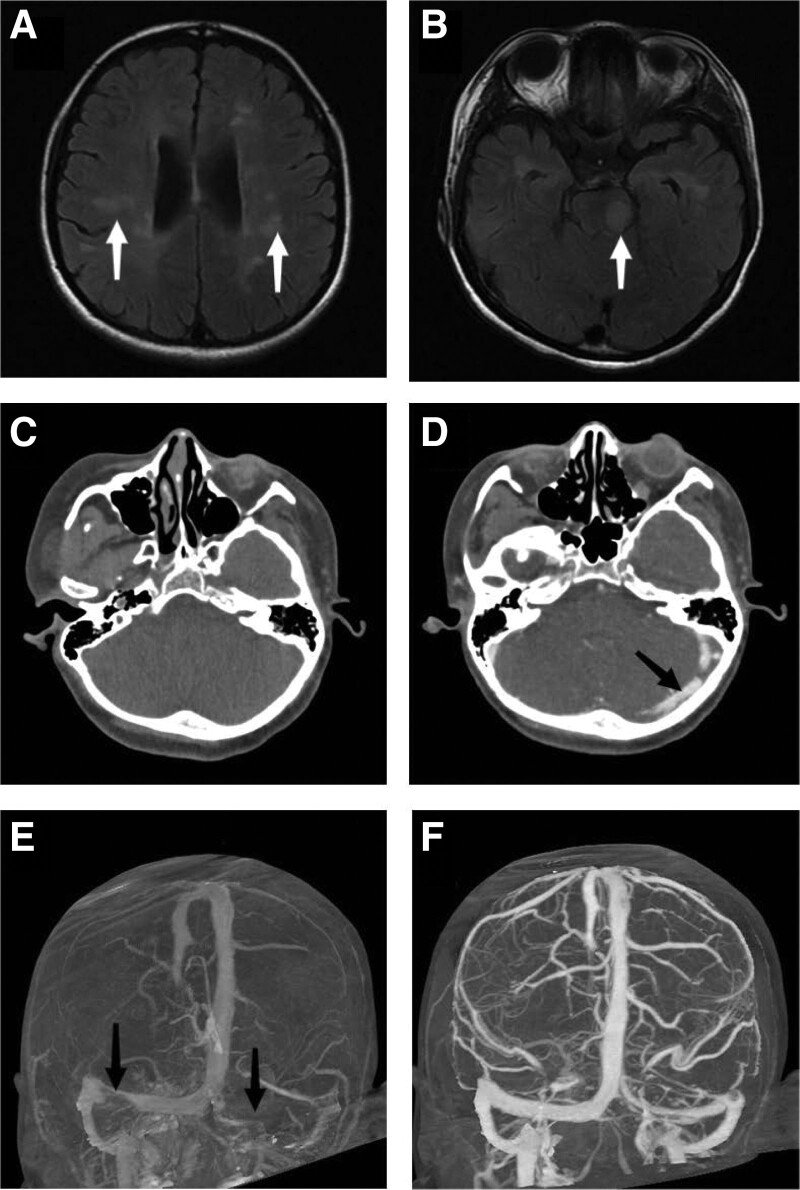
(A and B) Axial FLAIR magnetic resonance image demonstrates hyperintense. MS lesions in the pons. (C) Axial Cranial CT image without a signal change suggesting cerebral venous thrombosis on admission. (D) After 8 days of high-dose steroid treatment, cranial CT demonstrates the left sigmoid sinus, transverse sinus, and confluent sinus was hyperdense. (E) Cephalic venous CT demonstrates thrombosis in the bilateral transverse and left sigmoid sinus. (F) After anticoagulation therapy, no cerebral venous thrombosis was found on cephalic venous CT at 5-mo follow-up. CT = computed tomography, FLAIR = fluid attenuated inversion recovery, MS = multiple sclerosis.
